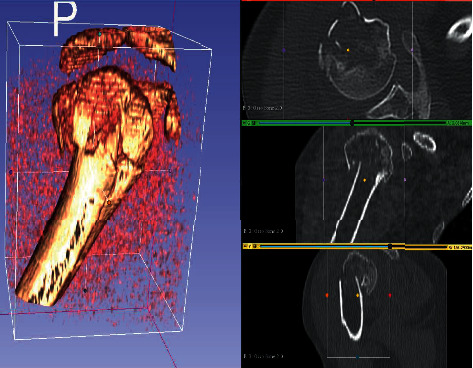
Slicer software user interface showing the segmentation process of a 3D digital model from a CT scan of PHF.
